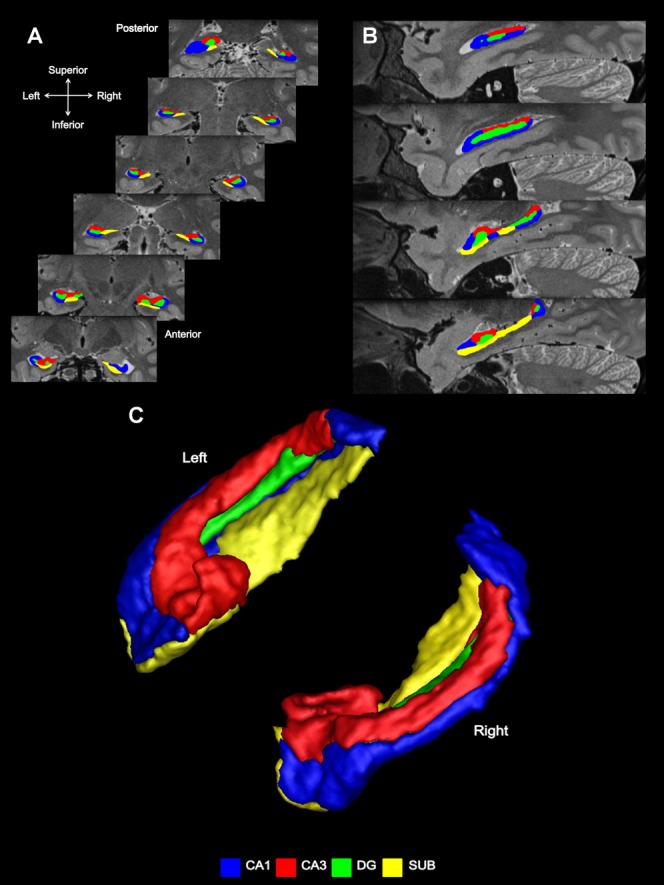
Subfield segmentation. (A) In the coronal plane—coronal sections through an averaged T2-weighted image of the left and right hippocampus of an example participant. (B) Subfield segmentation in the sagittal plane. (C) An example of subfield segmentation in 3D. [Color figure can be viewed in the online issue, which is available at http://wileyonlinelibrary.com.]
